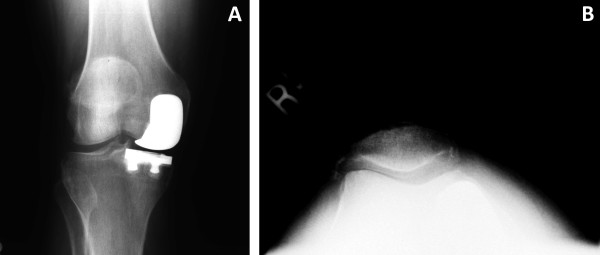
Antero-posterior (A) and Merchant view (B) radiographs of the same patient as Figures 1 and 2, taken at 41 month follow-up. The patient complained of increasing patello-femoral pain, and was revised to a total knee arthroplasty shortly thereafter.
